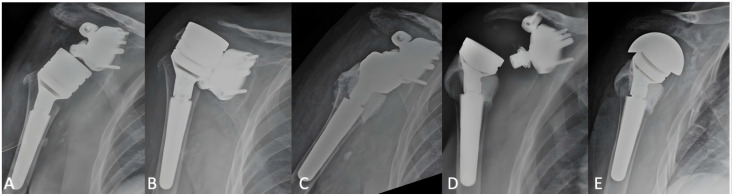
(A–E): Postoperative radiographs of the unstable reverse shoulder arthroplasty (RSA) described in Figure 3. (A) Anteroposterior view following revision with a metallic humeral spacer. (B) Dislocation observed two months after the revision. (C) Second revision procedure involving removal of the metallic spacer and cement filling of the proximal humeral metaphysis to increase the deltoid wrapping angle. (D) Dislocation noted three months after the second revision. (E) Final revision with conversion to hemiarthroplasty.
